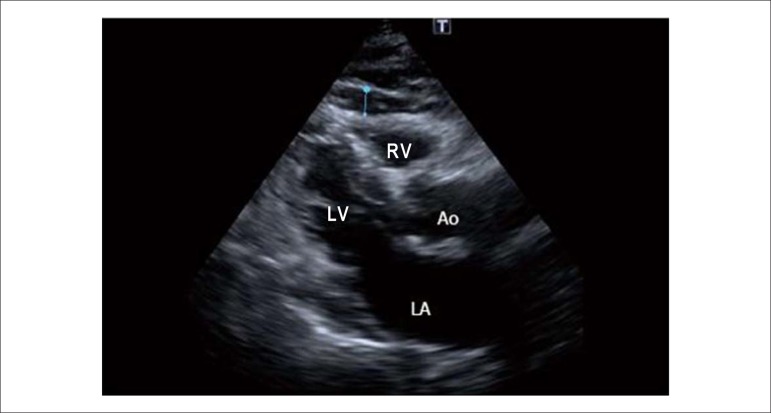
Measurement of epicardial fat thickness by two-dimensional transthoracic
